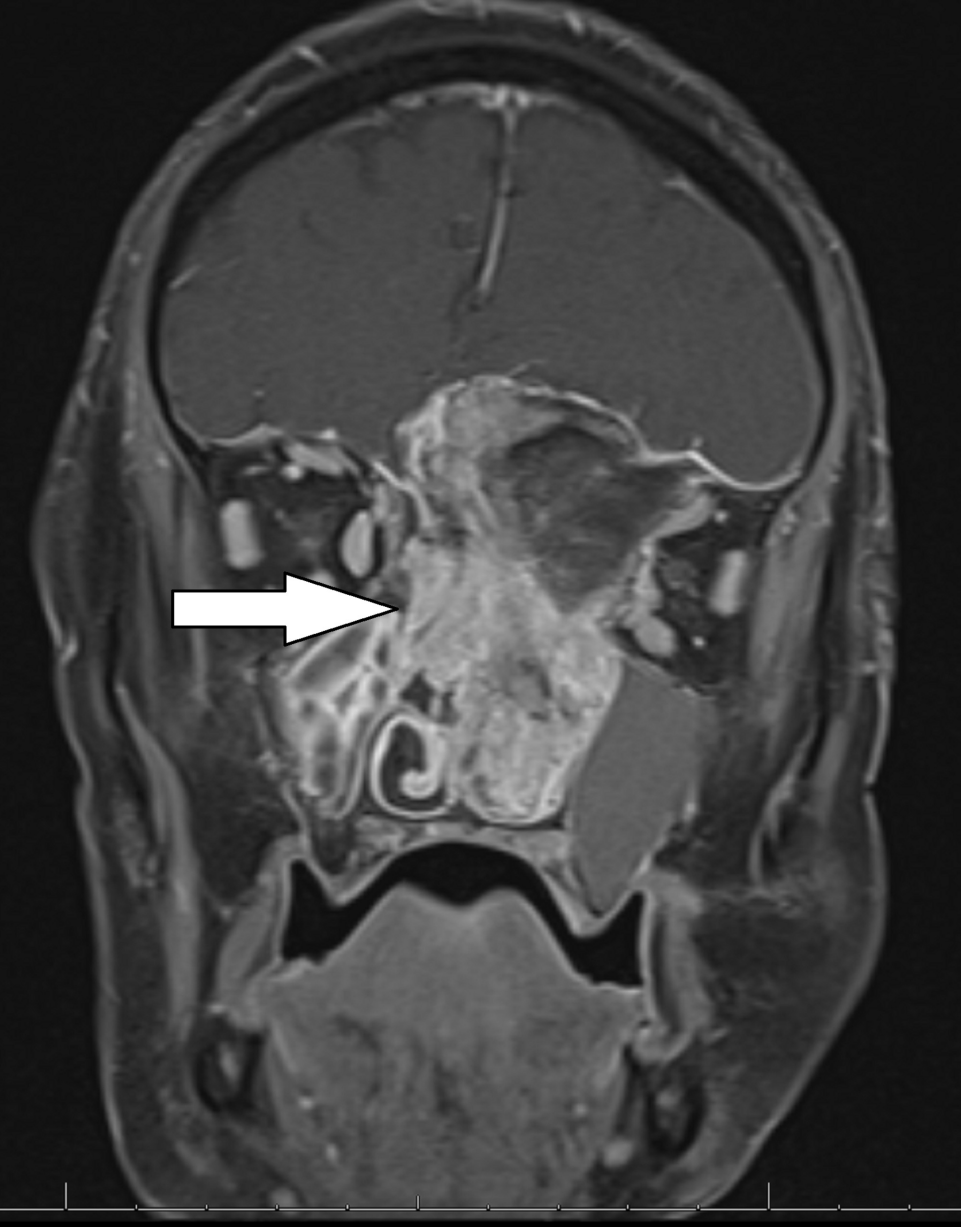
T1 weighted post-contrast coronal MRI
